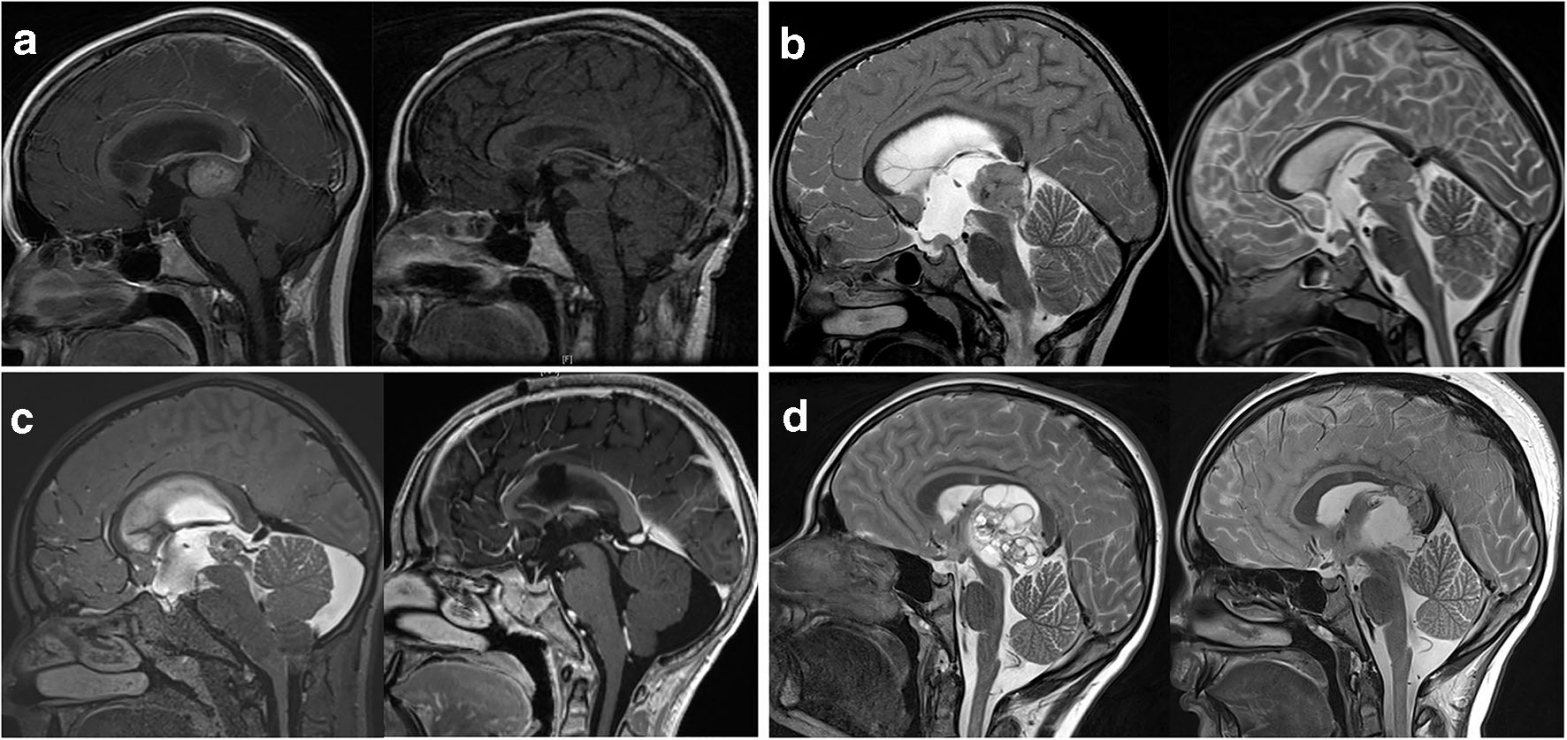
Representative MR images. a Papillary tumor of the pineal region, treated with ETV and biopsy, microsurgical supracerebellar infratentorial resection, radiation, and chemotherapy (outcome: stable disease). b Disseminated pineoblastoma with involvement of the anterior third ventricular floor, treated with stented ETV and biopsy (right image demonstrating the stent in place though the floor of the third ventricle), radiation, and individualized poly-chemotherapy (outcome: death). c Pineoblastoma, treated with ETV and biopsy, neoadjuvant chemotherapy, and transcallosal microsurgical resection, followed by radiation and chemotherapy (outcome: stable disease). d Mixed malignant germ cell tumor, transferred after primary biopsy (external hospital), treated by transcortical, transventricular microsurgical resection; radiation; and chemotherapy (outcome: complete remission)
